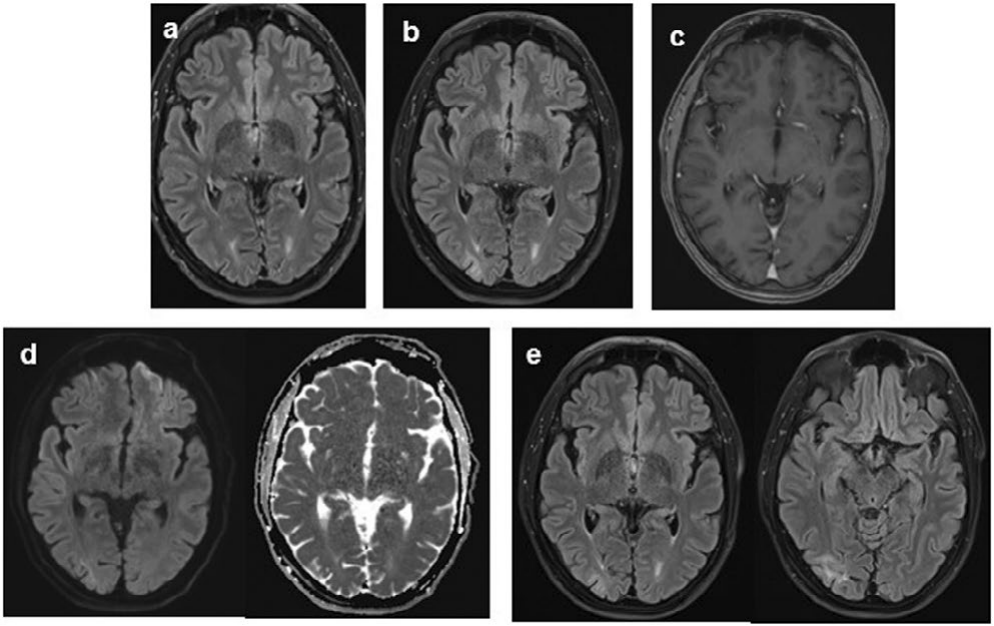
FIGURE 2 Case 2. Baseline brain MRI (a). Hyperintense right occipital juxtacortical lesion (b), with no contrast enhancement (c) and mild peripheral diffusion restriction on DWI (d). Stability on follow‐up MRI (e).
